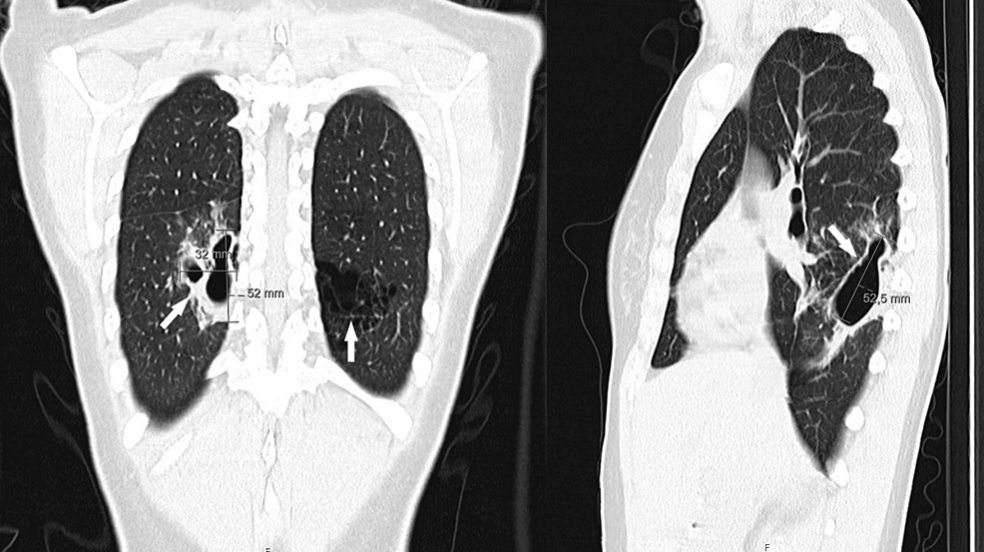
Chest CT scan showing two adjacent cavitations with thick walls on the right lower lobe
